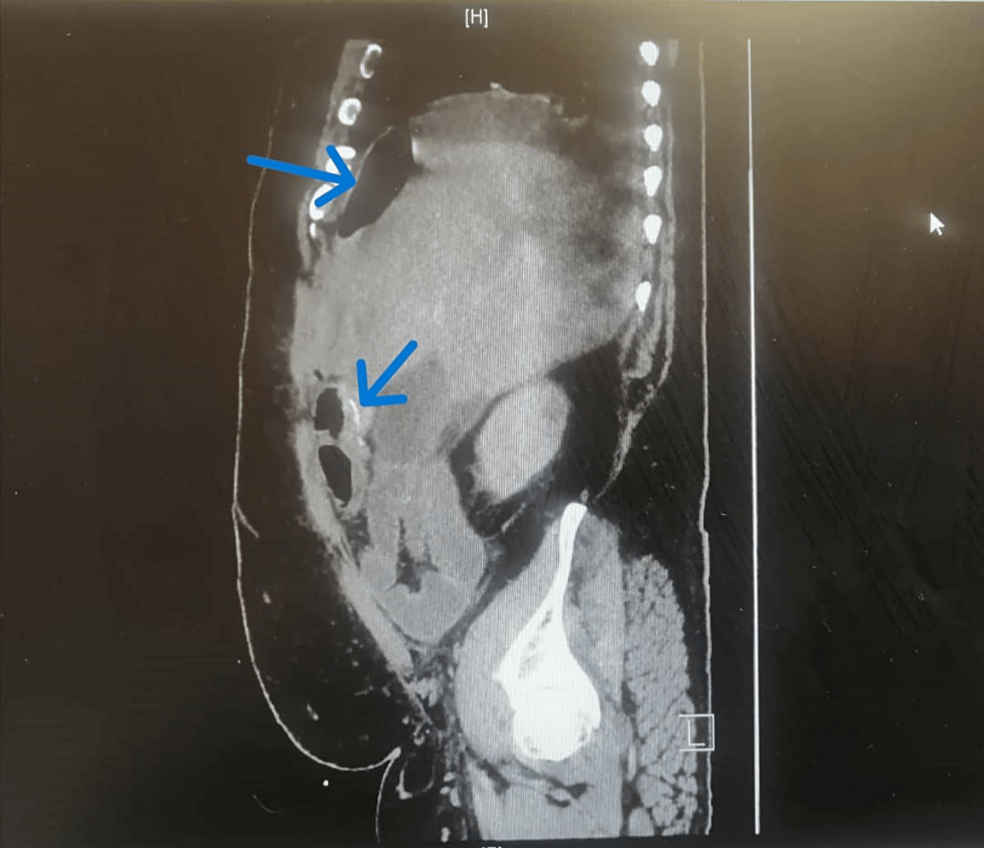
CT of the abdomen and pelvis with oral and IV contrast on POD 7 showing increased pneumoperitoneum, suggesting perforation with features of peritonitis. The ileocolic anastomosis appears unremarkable. POD: Postoperative day.
